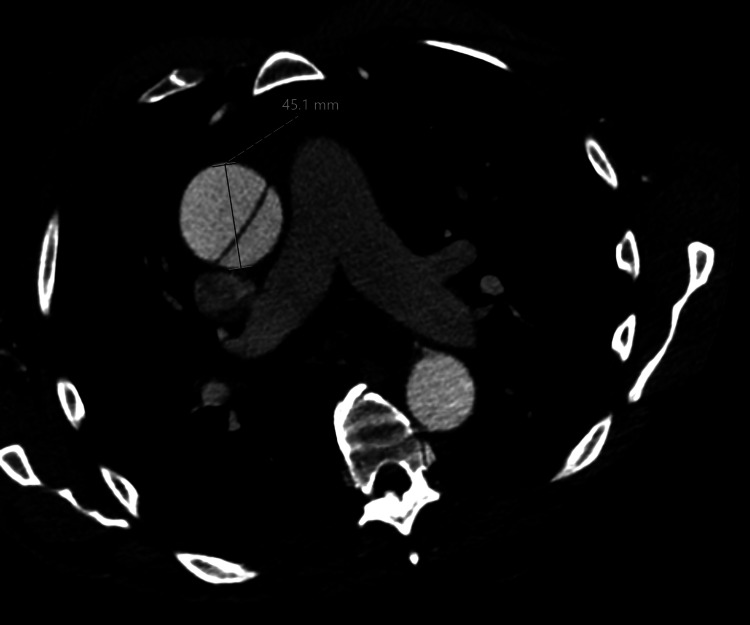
Aortic dissection at the aortic root on CTA. CTA: computed tomography angiography
